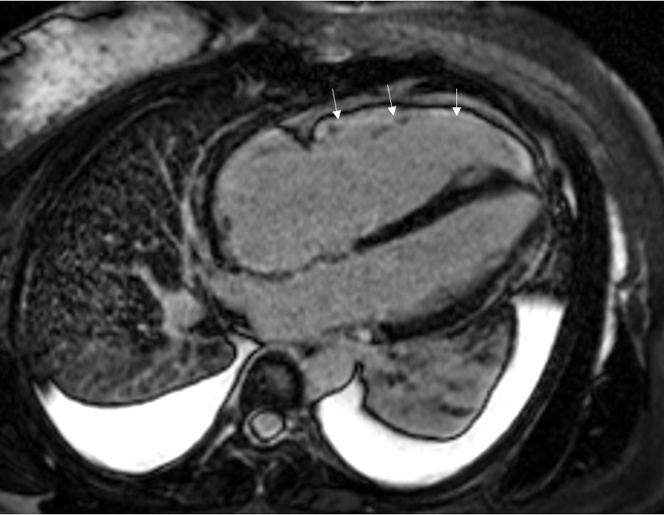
4-Chamber Delayed Enhancement Cardiac MRI Image demonstrating diffuse late gadolinium enhancement of the lateral right ventricular wall. Note right atrial and right ventricular enlargement and small pleural effusions. The arrows indicate the areas of late gadolinium enhancement. MRI = magnetic resonance imaging.
